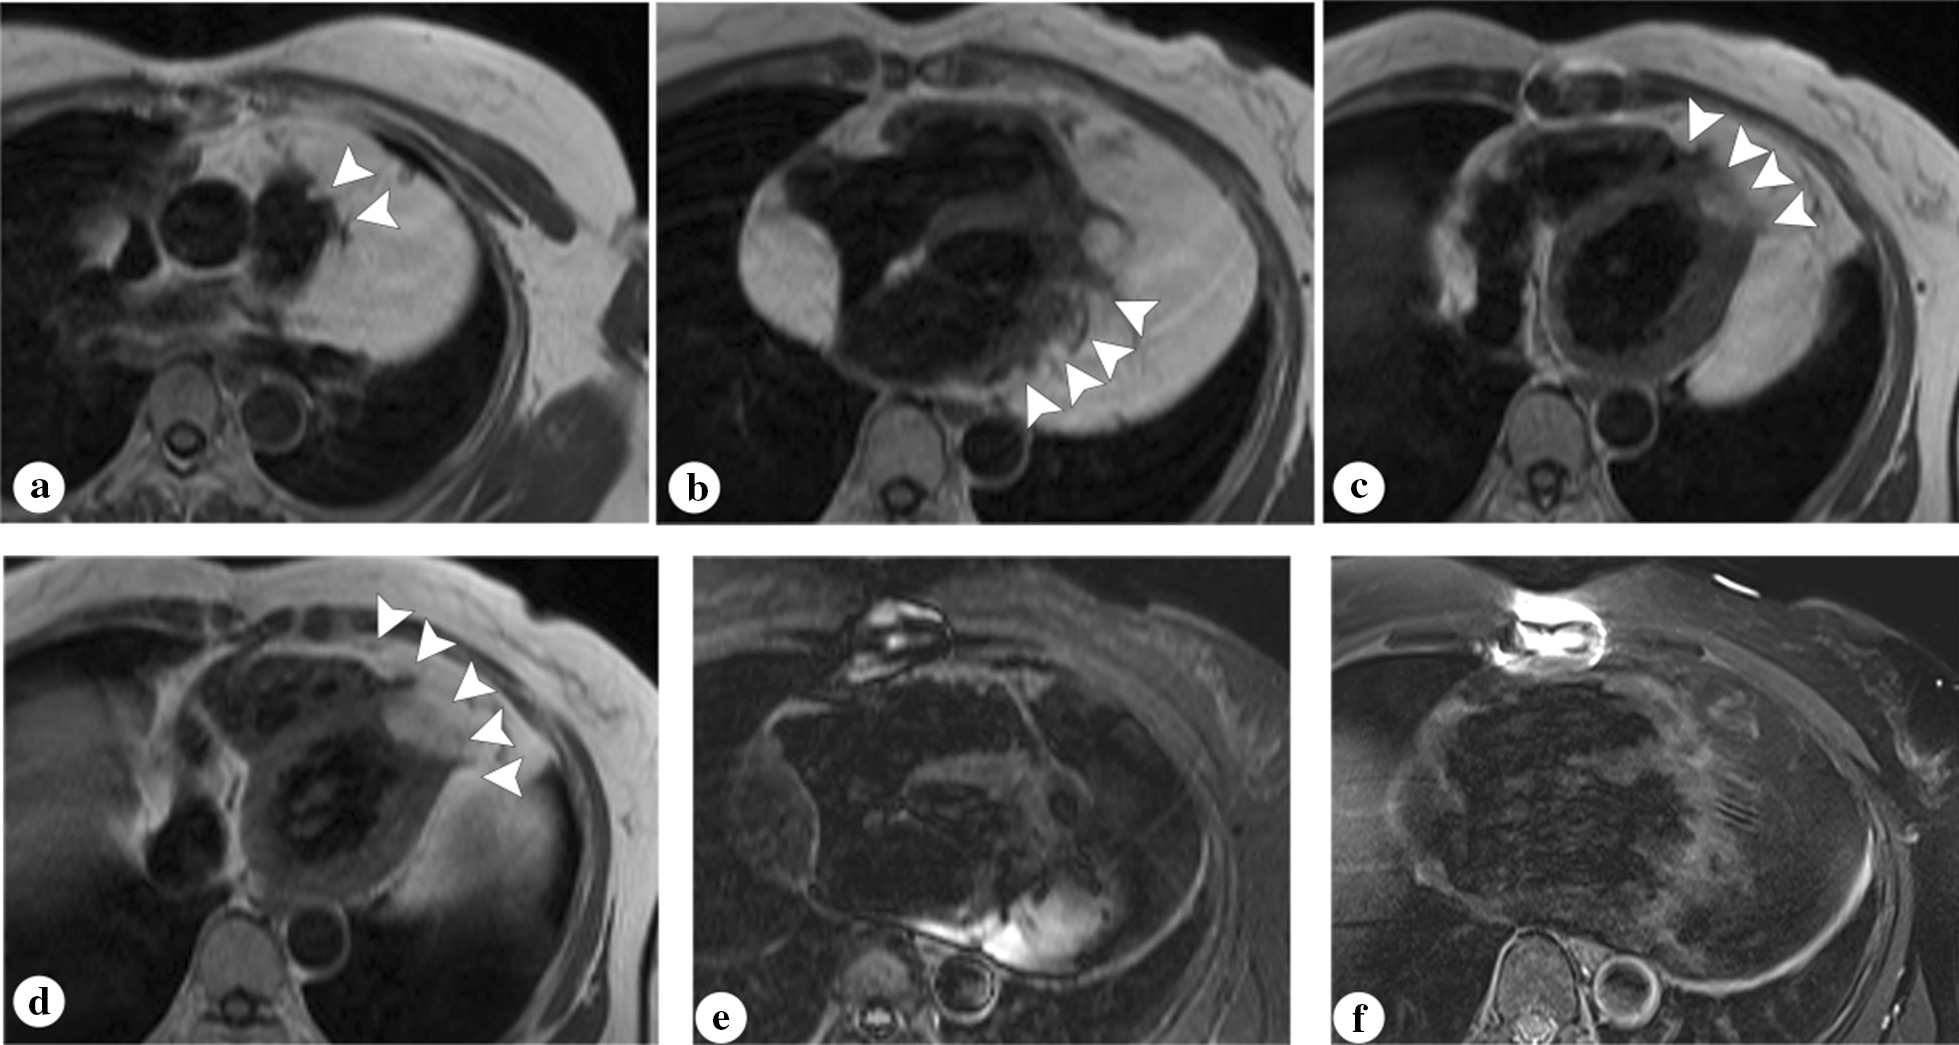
MR images of a giant pericardial lipoma of a female patient with history of pericardial lipoma resection. The T1 weighted (a, b, c, d) and T2 weighted fat suppression (e, f) images confirmed the diagnosis of lipoma. Diffuse infiltration of the lipoma to pulmonary arterial wall (a) and ventricular myocardium (b, c, d) was clearly observed (arrowhead)
